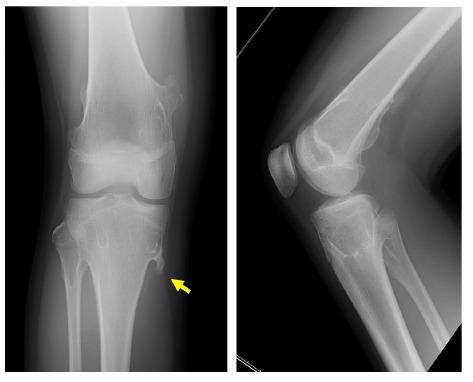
A 15-year-old girl with hereditary multiple exostoses. Plain radiographs show multiple protruding lesions arising from the distal femur, the proximal tibia and the proximal fibula (left: antero-posterior view, right: lateral view). (A yellow arrow indicates the tip of the osteochondroma causing the pain).
